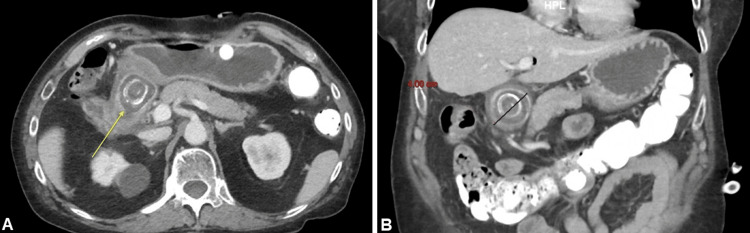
(A) Yellow arrow pointing to the initial axial CT of the abdomen, showing a large 4 cm gallstone impacted in the gastric outlet. (B) Initial coronal CT of the abdomen showing the same gallstone impacting the gastric outlet.
